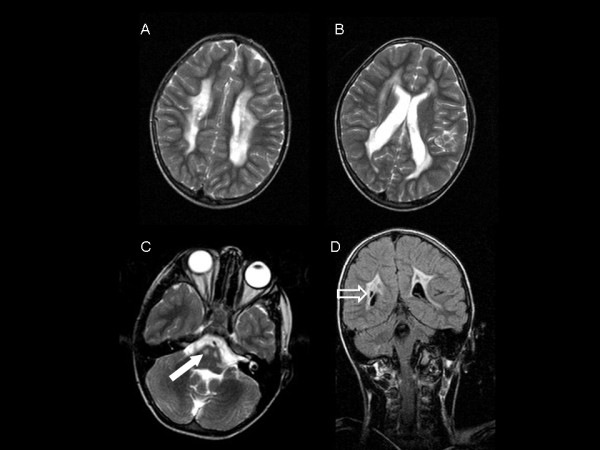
(A-C) Axial T2-weighted and (D) coronal FLAIR-weighted MR images of patient 3 at 9 years of age show widespread bilateral T2-hyperintensities in cerebral periventricular white matter. Involvement of the pons (C, full white arrow) and cystic lesions (D, open arrow) are visible. Peripheral U-fibers are spared. Lesions are largely unchanged compared to neuroimaging at age 4 years^1^.
